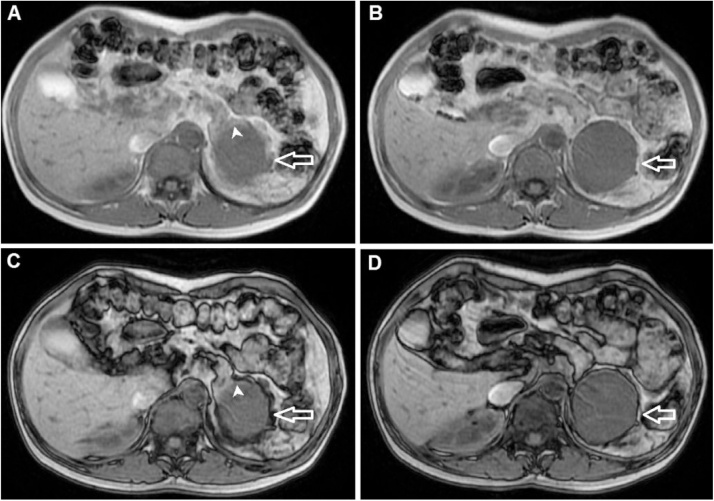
Axial in-phase T1-weighted images (A and B) and axial opposed-phase T1-weighted images (C and D). Axial T1-weighted images showed that the mass had low signal intensity compared to liver parenchyma (A and B, arrow) and did not lose signal intensity on T1 opposed-phase images (C and D, arrow). Part of the mass was suspected to be connected to the pancreas tail (A and C, arrowhead).
